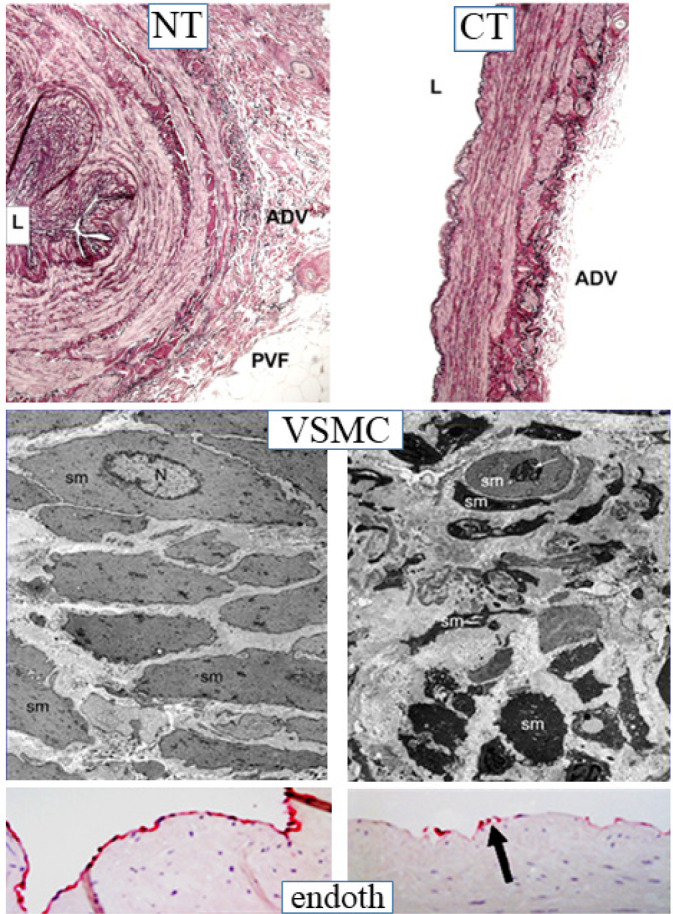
No-touch (NT) versus conventional (CT) saphenous vein (SV); vascular
